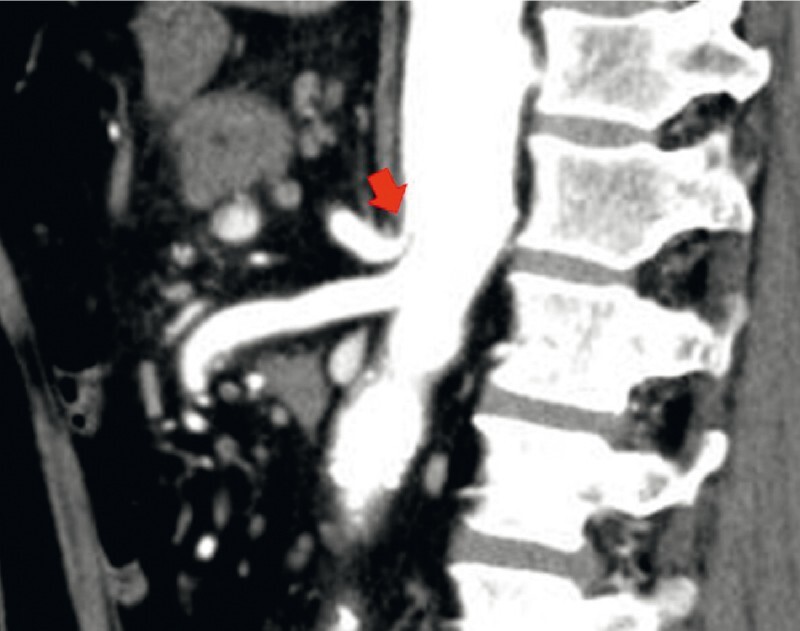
Contrast-enhanced computed tomography scan image showing celiac artery stenosis due to the median arch ligament (arrow).
